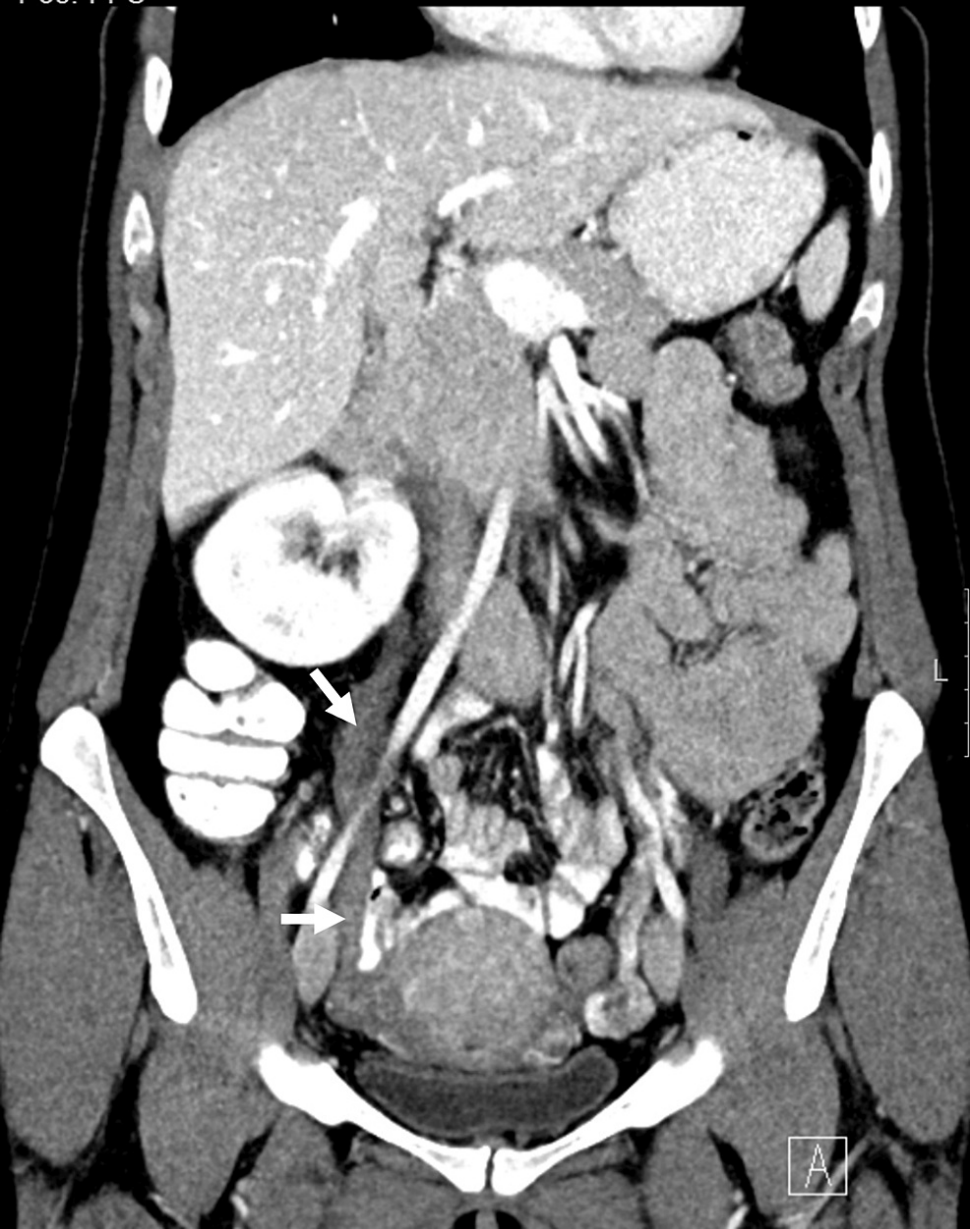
Post-contrast CT image (coronal) Coronal reformatted post-contrast CT image demonstrates distended thrombosed right ovarian vein (white arrows) in a 32-year-old female at day 6 post-cesarean section. This patient was treated by the authors at King Abdulaziz University Hospital.
